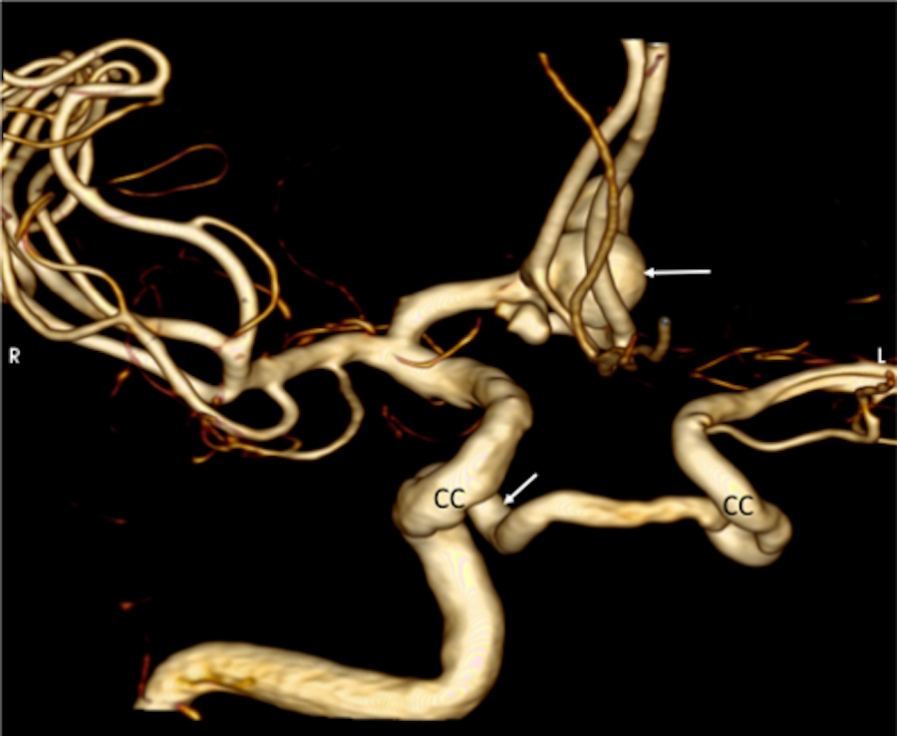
3D reconstruction from a CT angiography, noting the anterior circulation aneurysm (upper arrow) and the origin of the left internal carotid artery from the right cavernous segment (CC) of the right internal carotid artery (lower arrow).
